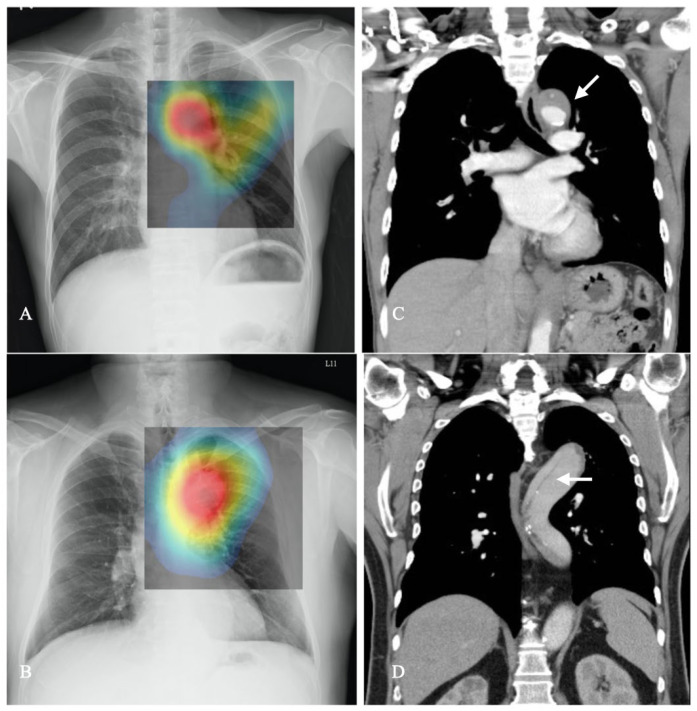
The images on the left (A,B) show the location of a possible aortic lesion on the CXR marked by the CNN model (VGG19), and these areas are colored by CAM to make it easy for users to interpret them. The pictures on the right (C,D) are the CTA images of the same patient showing the true location of the patient’s aortic lesion. After comparing with the CAM image, it was found that the CNN model correctly interpreted the location of the lesion on the CXRs, arrow: false lumen of aortic dissection.
